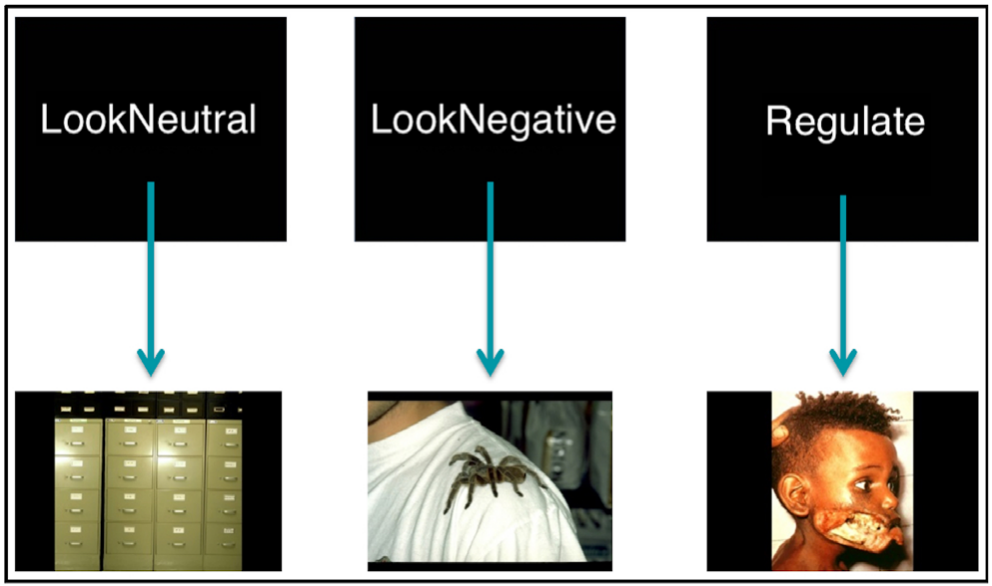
Functional MRI task example images for LookNeutral, LookNegative, and Regulate conditions. Participants were presented with neutral and negative images and were instructed to either (1) LookNeutral or passively observe neutral images; (2) LookNegative or maintain emotions elicited by negative images; or (3) Regulate or reappraise negative emotions elicited by negative images. [Color figure can be viewed at wileyonlinelibrary.com]
